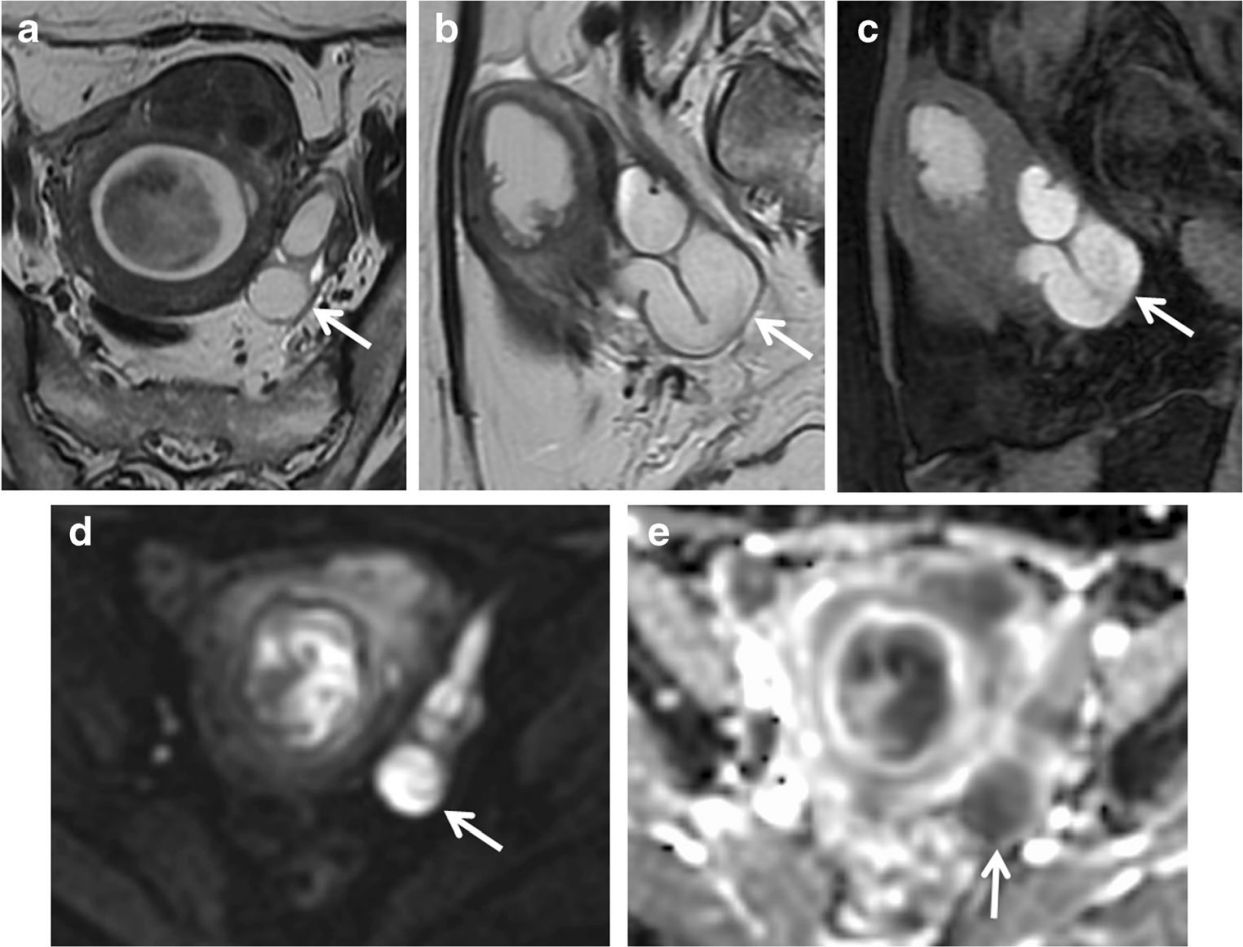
Hematosalpinx in a 62-year-old woman with cervical carcinoma. (a) Axial and (b) sagittal T2-weighted images show a serpentine fluid-filled left adnexal structure with internal high to intermediate signal intensity (*white arrows*). The endometrial cavity is distended by high to intermediate signal intensity content. (c) Sagittal fat-suppressed T1-weighted image show a distended fallopian tube with high signal intensity content (*white arrows*), a finding consistent with hematosalpinx. (d) Axial DW image (b = 800 s/mm^2^) and (e) corresponding ADC map show that the tubaric content demonstrates restricted diffusion with high signal intensity (*white arrow*) on DWI image and low signal intensity (*white arrow*) on ADC map
